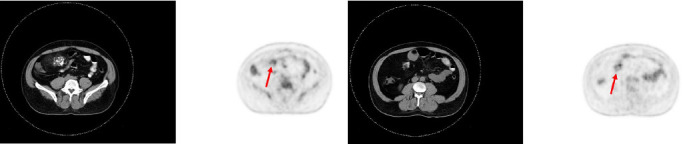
(a, b) CT scan of the abdomen juxtaposed with corresponding PET scan.
